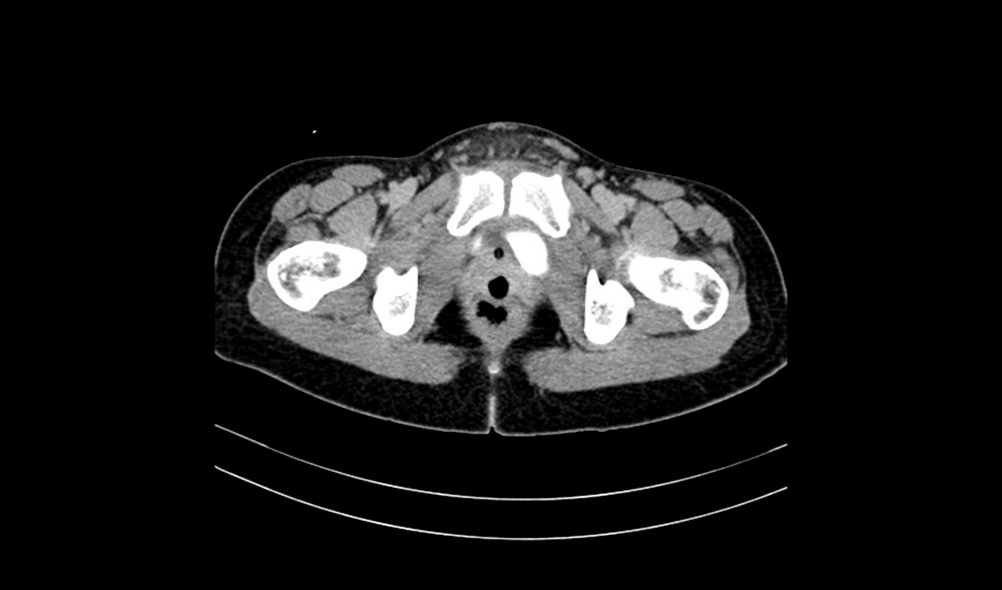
Delayed post-intravenous contrast CT scan (axial reformat) showing the presence of a hypodense vaginal tampon and the absence of a ureterovaginal fistula for case 2.
